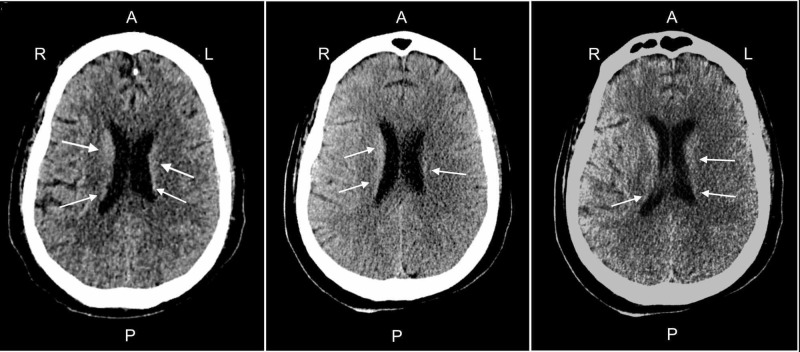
Head CT without contrast from different angles. Blood pooling in the anterior aspect of the lateral ventricles into the occipital horns bilaterally. A - Anterior P - Posterior R - Right L - Left
